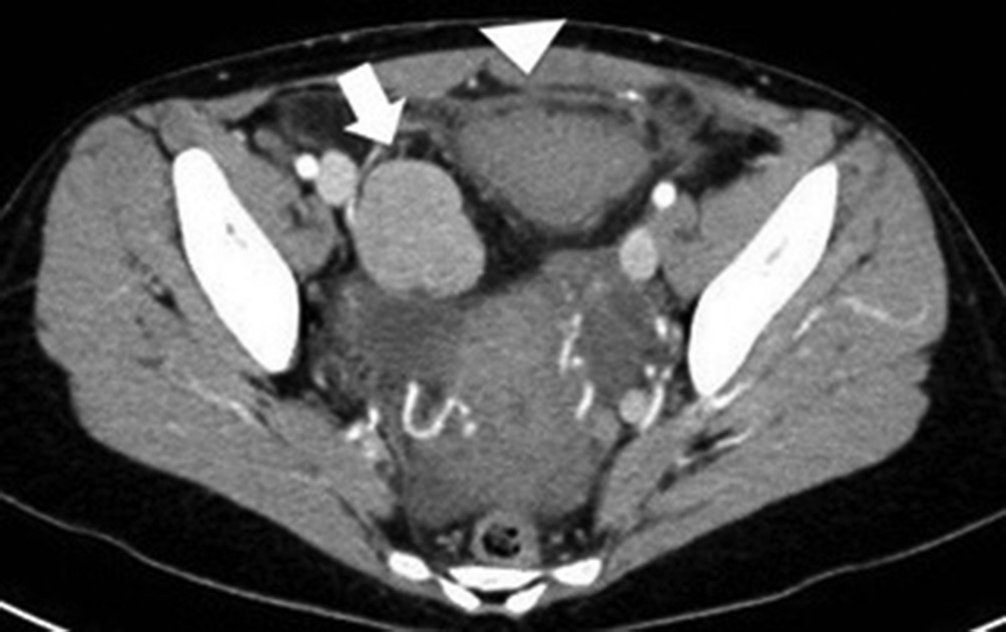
Axial contrast-enhanced CT scan shows poor contrast enhancement of the left splenic implant surrounded by fat stranding and a small amount of fluid in the recto-uterine pouch, in keeping with torsion (arrow head). There is a right contrast enhancing pelvic implant as well (arrow).
